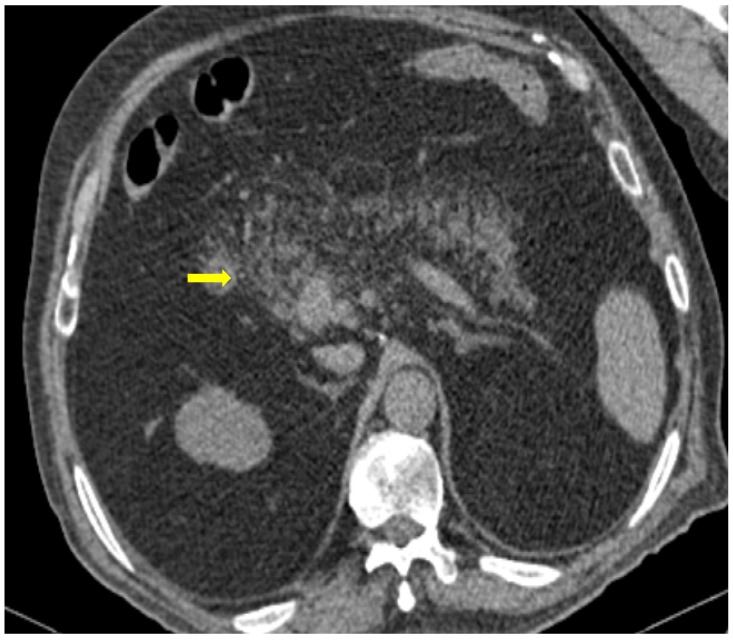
81-year-old patient with COVID-19 pneumonia developed epigastric pain at the second day of hospitalization. CT demonstrating acute pancreatitis (yellow arrow).
